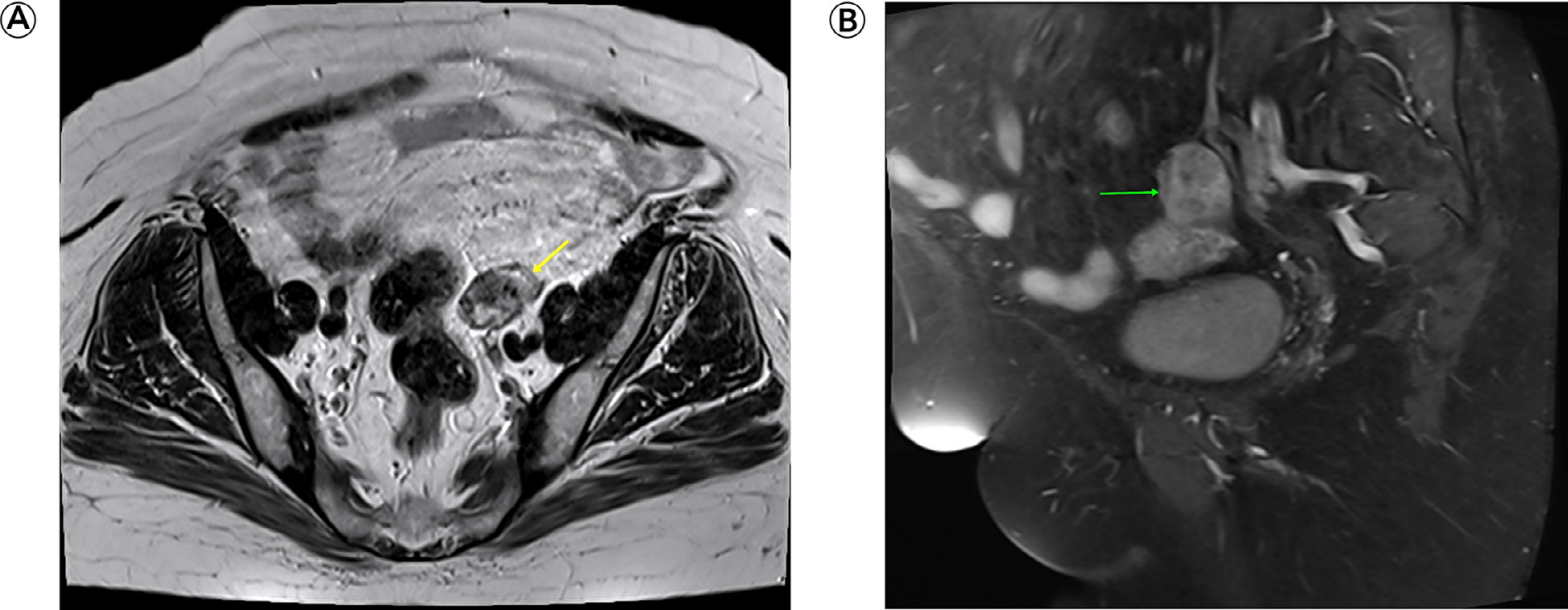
Pelvic MRI. (A) Axial T2 section shows a mass of heterogeneous intensity in the left ovary (yellow arrow) (B) Contrast-enhanced T1 fat suppression shows a heterogeneously enhancing mass (green arrow).
